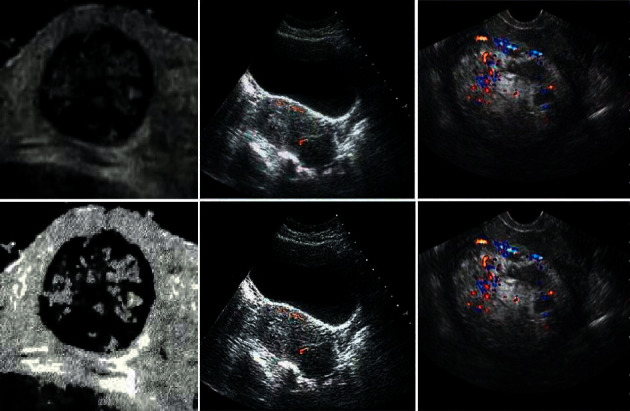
Comparison of ultrasonic noise reduction images before and after the algorithm.
